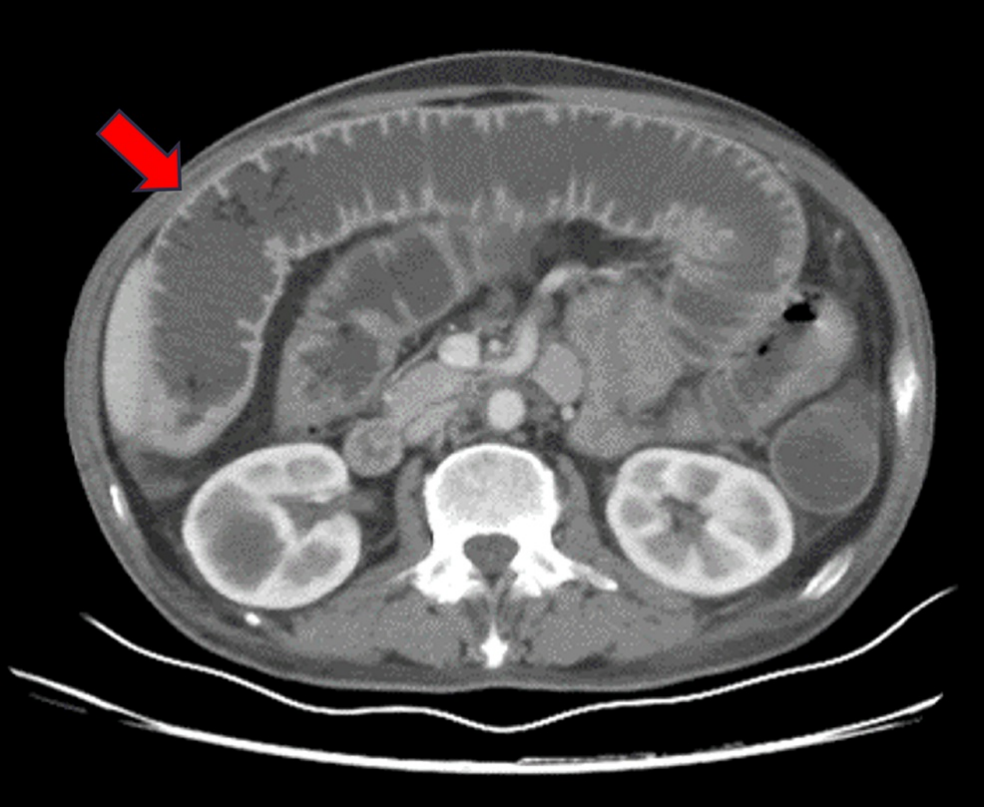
Contrast-enhanced computed tomography revealed a short segment dilatation of the jejunal loop with loss of valvulae conniventes (red arrow). No mesenteric vessel thrombus was noted.
